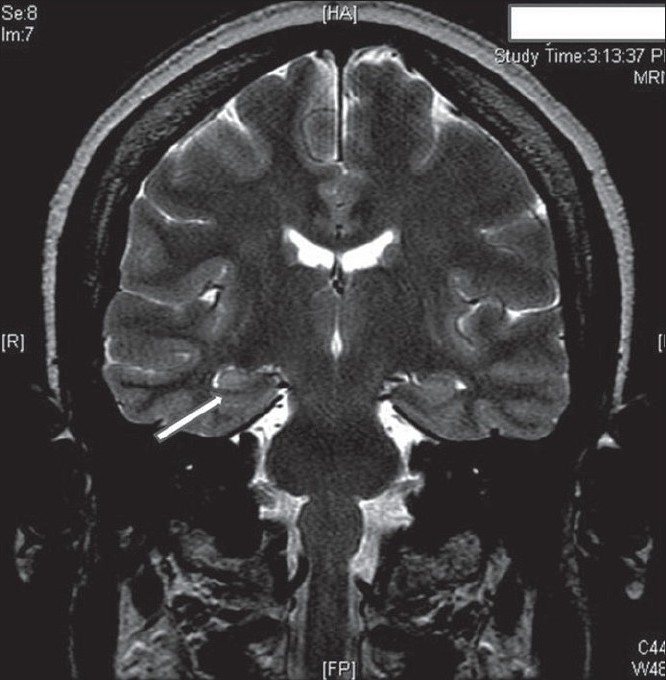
1.5T MRI: T2-weighted coronal view, showing right hippocampal sclerosis (arrow)
